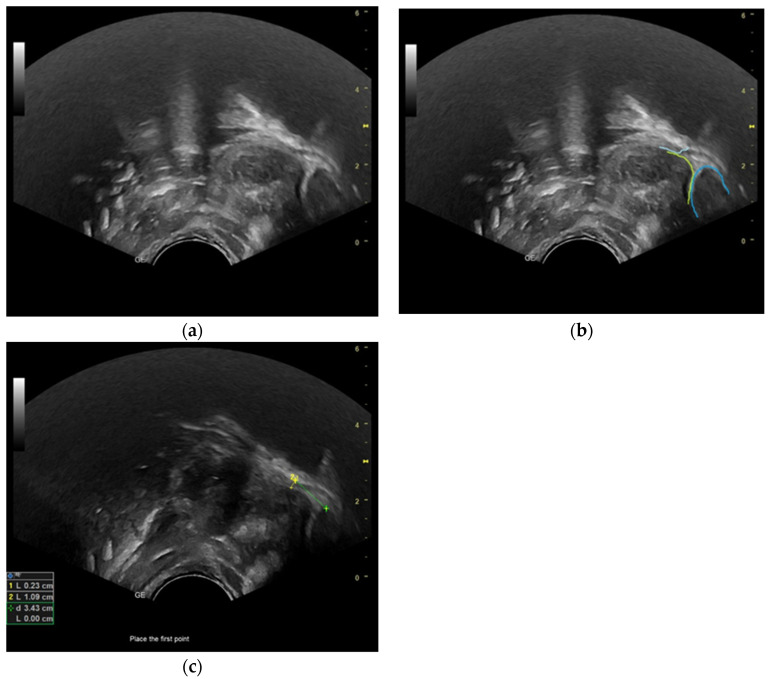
Ultrasonographic image of breastfeeding: (a) nipple view, tongue up; (b) nipple: dark blue line, hard–soft palate junction (HSPJ): light blue line, tongue: yellow line; (c) depth of intraoral space, cm: yellow line 1; nipple–HSPJ distance, cm: green line 2.
